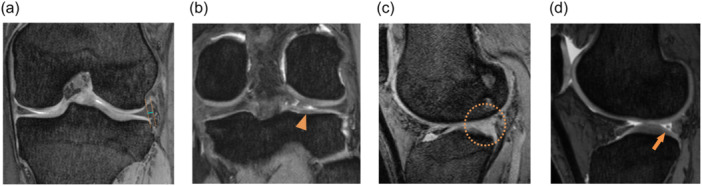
Magnetic resonance imaging (MRI) signs for a lateral meniscus posterior root tear in the left knee. (a) The lateral meniscus extrusion (LME) is evaluated on the coronal MRI slice showing the maximum extrusion of the lateral meniscus. The LME width is defined as the distance (indicated with a blue line) from the most peripheral aspect of the lateral meniscus (LM) to the border of the tibia (both indicated with orange lines). The lines are parallel to the outer margins of the femur and tibia, excluding any osteophytes. (b) On coronal images, the cleft sign is defined as a vertical linear defect around the meniscal root (indicated with an arrowhead). (c) On a sagittal slice, the ghost sign is defined as the absence of the entire contour of the LM (surrounded by a circle). (d) On a sagittal slice, the truncated triangle sign is an abrupt termination of the normal triangular meniscal contour at its tip (indicated with an arrow).
